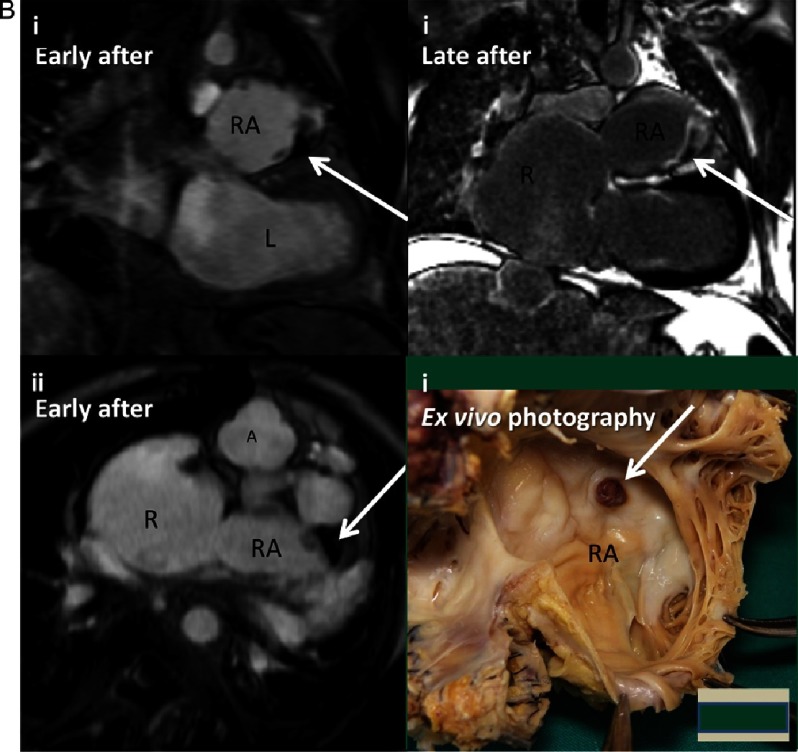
(A) Patient 2 imaging findings. (i) Chest radiography in posteroanterior view. As for patient 1, left juxtaposition of the atrial appendages produces a bulging left heart contour (white arrows). Situs solitus, cardiomegaly and scoliosis are also noted. (ii) Corresponding coronal image from 3D bSSFP for direct comparison with 2Ai. The left juxtaposed right atrial appendage (white arrows) appears almost of the same size as the already dilated right atrium. (iii) Right atrial dilatation with sluggish blood flow on still frame from cine CMR, axial view, similar to the findings for patient 1. The arrow points to the surgical patch to close off the right atrial to right ventricle connection. (iv) 3D bSSFP sagittal image showing dilated right atrial appendage (RAA) towards the left side. Underlying transposition of great arteries can be noted with anterior aorta from the RV. CMR, cardiovascular magnetic resonance; RA, right atrium; RAA, right atrial appendage; Ao, aorta; PA, pulmonary artery; LPA, left pulmonary artery; RV, right ventricle; LV, left ventricle; LA, left atrium. (B) Contrast-enhanced CMR and ex-vivo findings documenting intra-auricular thrombus in patient 2. Early after gadolinium injection, coronal (i) and axial (iii) images show, as for patient 1, a dark thrombotic region at the tip along the outer wall of the right atrial appendage (white arrows). The corresponding late gadolinium coronal image is again consistent with intra-auricular thrombosis (white arrow). Ex-vivo evaluation after explant for heart transplant (iv) confirmed the presence of a thrombus (white arrow). CMR, cardiovascular magnetic resonance; RA, right atrium; RAA, right atrial appendage; Ao, aorta; LV, left ventricle (Courtesy of Professor Siew Yen Ho).
